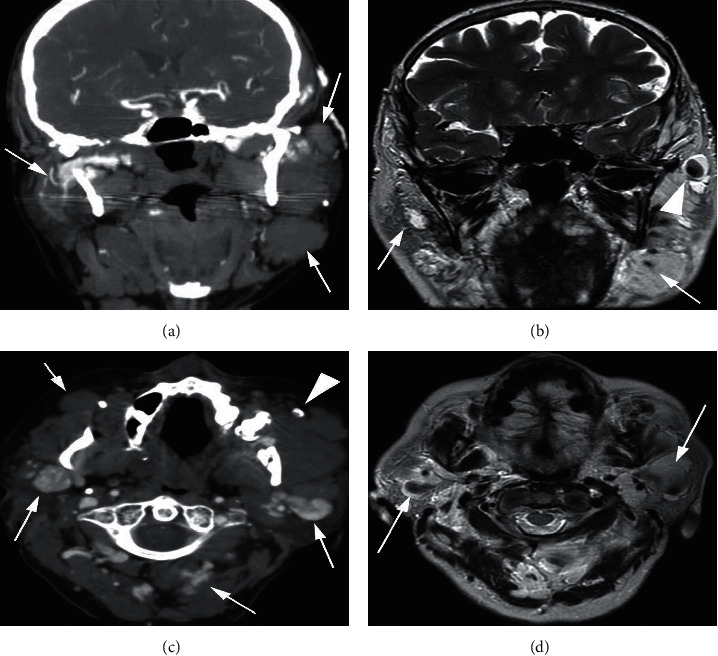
Contrast-enhanced CT ((a) coronal; (c) axial) and MRI (T2-weighted; (b) coronal; (d) axial). Note the numerous well-defined soft-tissue densities in the upper neck, which represent dilated, low-flow, venous channels (solid arrows, a–d). To avoid clutter, only some are pointed out. Calcified phleboliths are also notable within the dilated venous channels, which are commonly seen in low-flow venous malformations (arrow heads, b, c).
